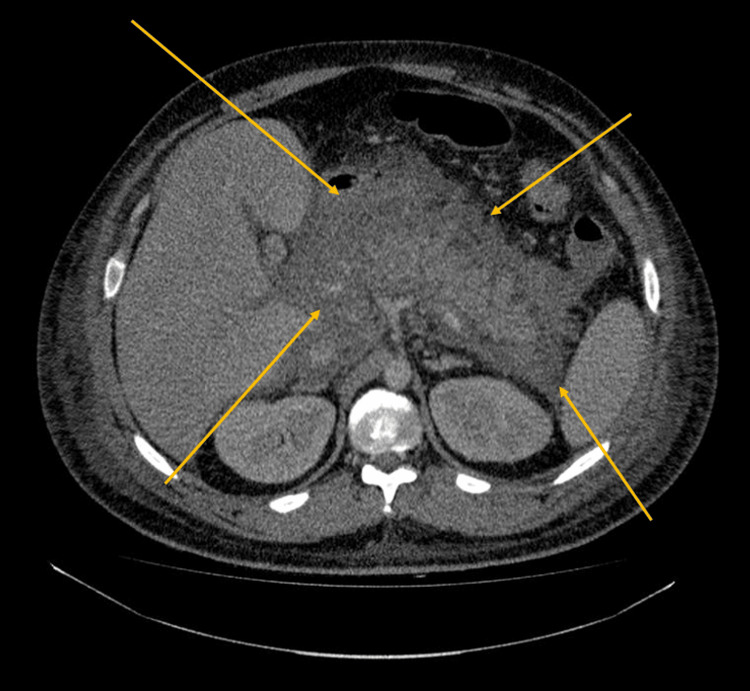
CT scan of the abdomen of Case 1 showed evidence of acute pancreatitis without focal necrosis. The arrows highlight radiological features consistent with acute pancreatitis, including pancreatic enlargement and surrounding peripancreatic inflammatory changes.
